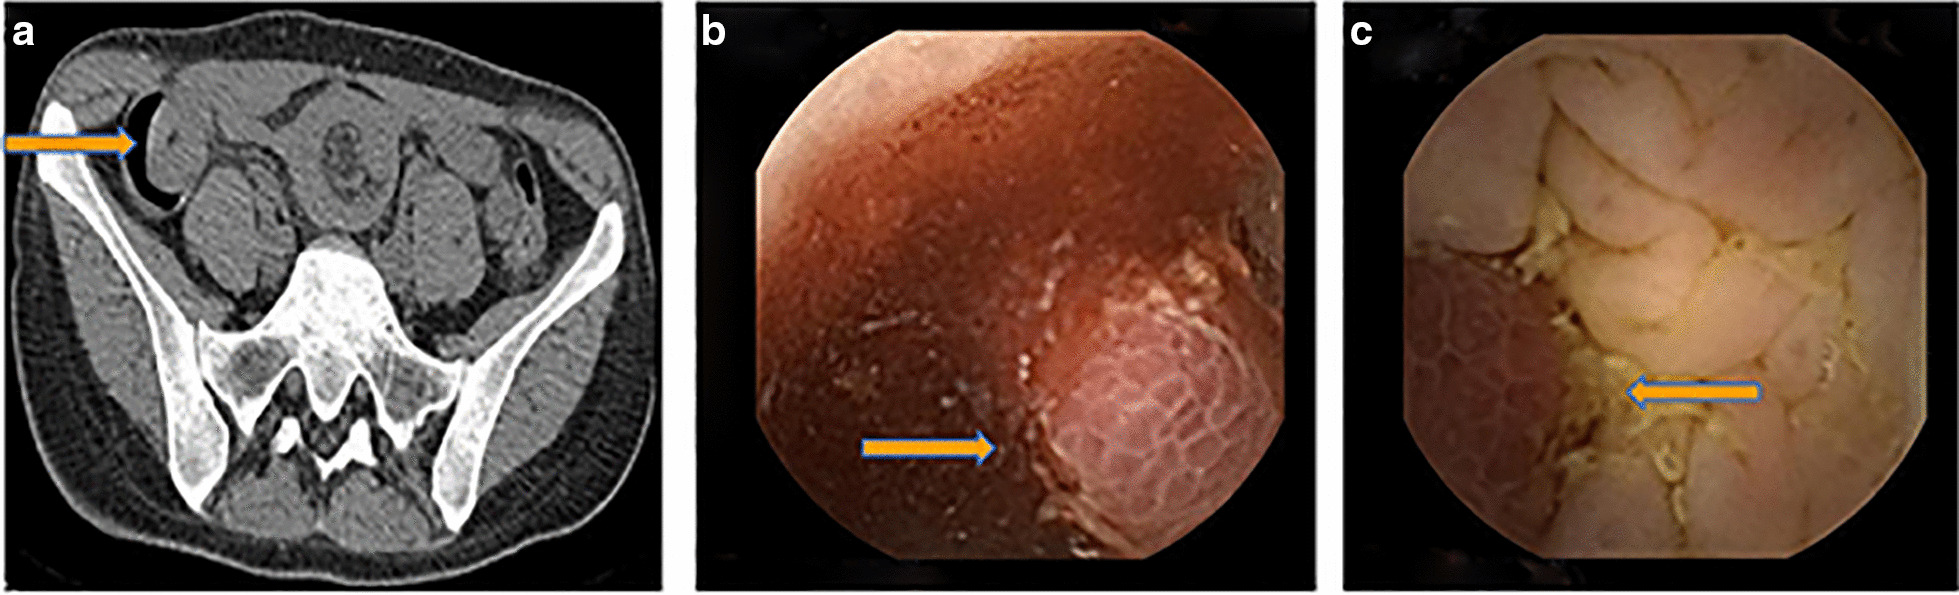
CT and capsule endoscope images of the lesion. Abdominal CT showed an indistinct ileocecal structure with a “doughnut” sign (yellow arrow) (a). Two subsequent capsule endoscopes revealed a protruding lesion with bleeding in the distal ileum(yellow arrows) (b, c)
